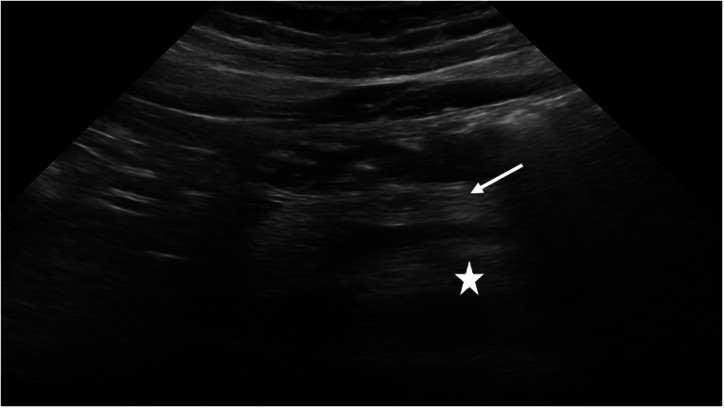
A representative image of pancreatic echogenicity in abdominal ultrasound. Grade 3: pancreatic echogenicity (thin arrow) is similar to or higher than the retroperitoneal fat (asterisk).
